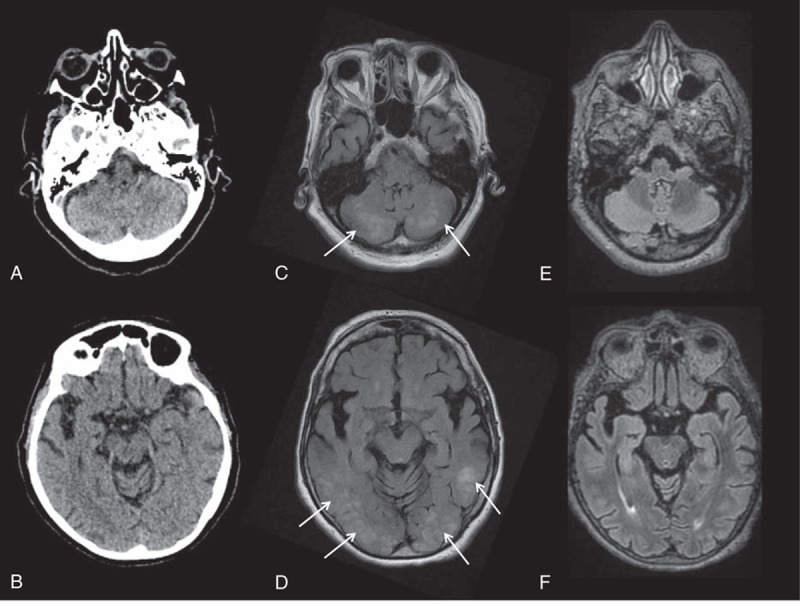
Cerebral imaging in a patient with duloxetine-related PRES. (Panels A and B) No abnormalities 5 days before the onset of PRES. (Panels C and D) FLAIR sequence showing bilateral high-signal foci in the occipital, parietal, and temporal lobes (white arrows). (Panels E and F) FLAIR follow-up sequence showing complete resolution of the abnormalities. FLAIR = fluid-attenuated inversion recovery, PRES = posterior reversible encephalopathy syndrome.
